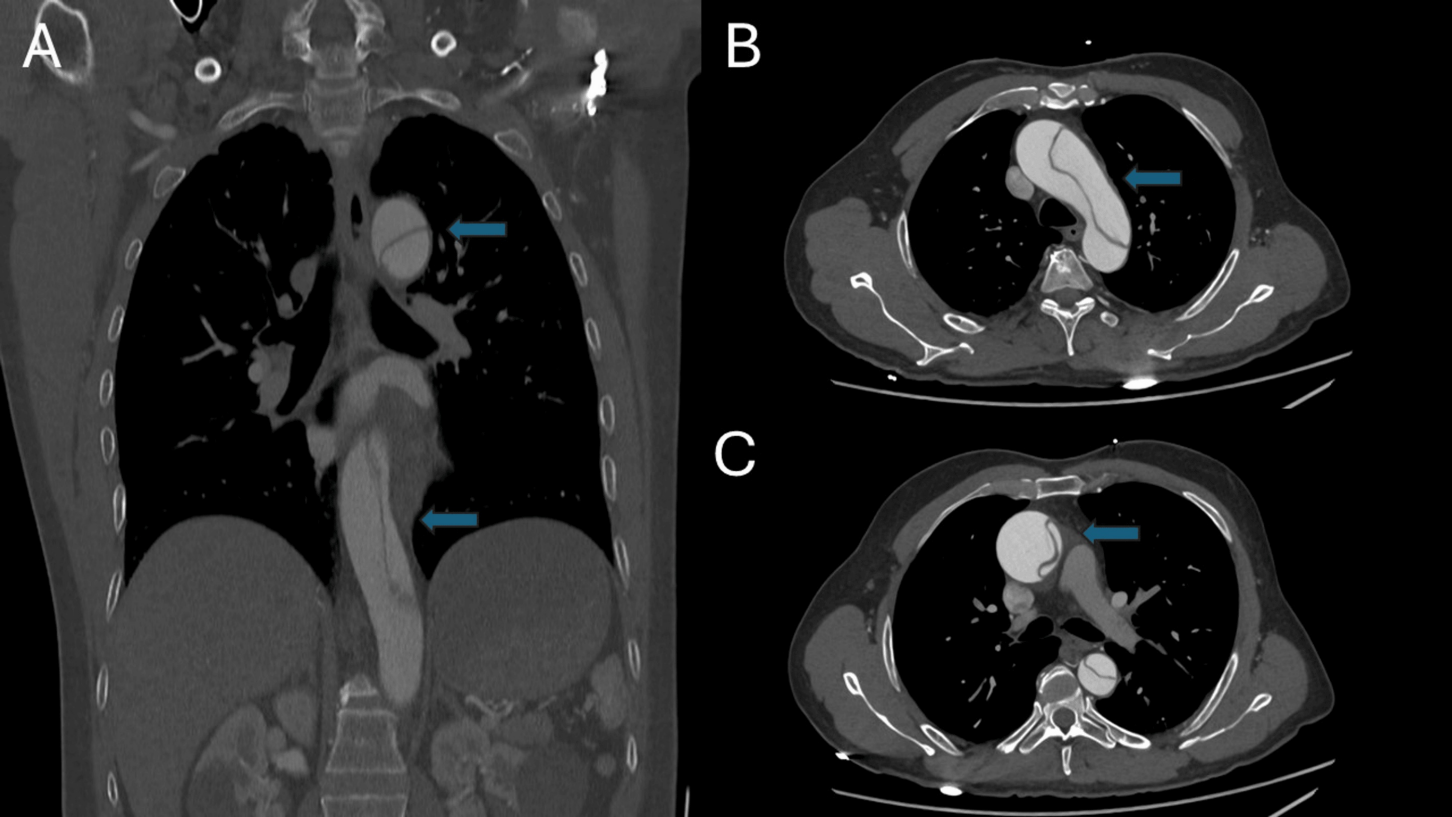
Multiple computed tomography angiography (CTA) scan slices showing extensive Stanford type A acute aortic dissection. The coronal image on the left (A) shows the extensive Stanford type A acute aortic dissection. Axial view (B) and (C) shows the intimal flaps. The intimal flap separates the true lumen (smaller caliber) from the false lumen (larger caliber) and is depicted by the blue arrows.
